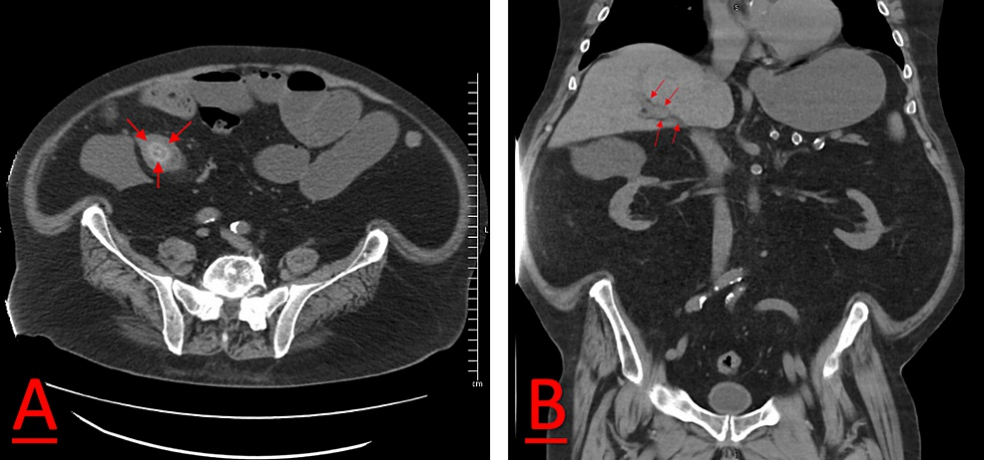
Axial and coronal CT scans demonstrating small bowel obstruction at the terminal ileum. (A) A 1.7 cm gallstone in the terminal ileum, which is causing obstruction, approximately 6 cm from the ileocecal valve (red arrow). (B) The patient’s gallbladder is not clearly seen on imaging, suggesting possible fistula between the gallbladder and the duodenum. There is a small amount of refluxed air on the extrahepatic bile ducts (red arrow). CT, computed tomography
